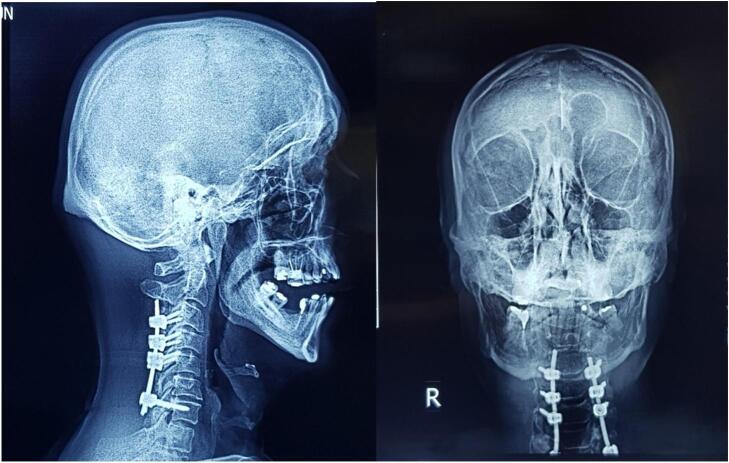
Sagittal (A) and coronal (B) X-ray radiographs of the patient's cervical spine following posterior cervical laminectomy and lateral mass screw/rod fixation from C3 to C7. The images show the alignment and positioning of the surgical hardware, providing an overview of the postoperative spinal stabilization.
